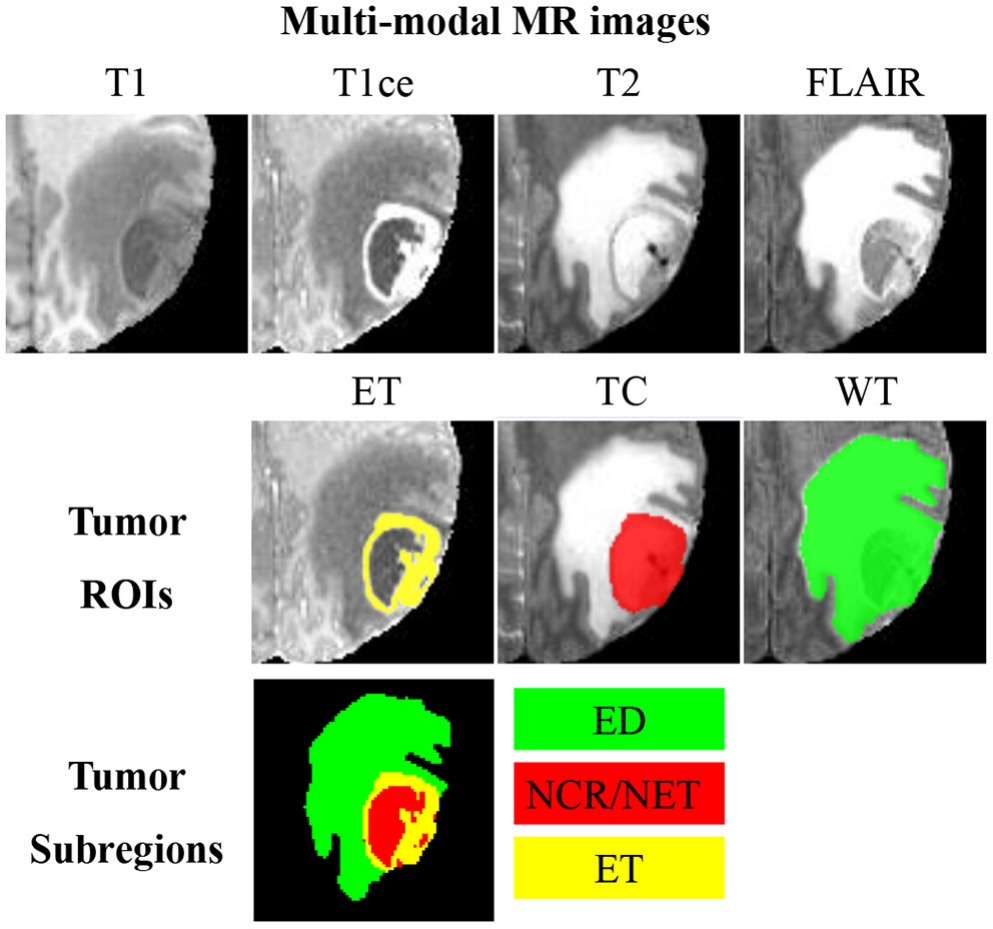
An example of multi-modal MR images, three partially-overlapping tumor ROIs (ET, TC, and WT), as well as three non-overlapping tumor subregions (ED, NCR/NET, and ET). MR images of different modalities can best display different ROIs; visualizations of ET, TC, and WC are, respectively, the best in T1ce, T2, and FLAIR.
